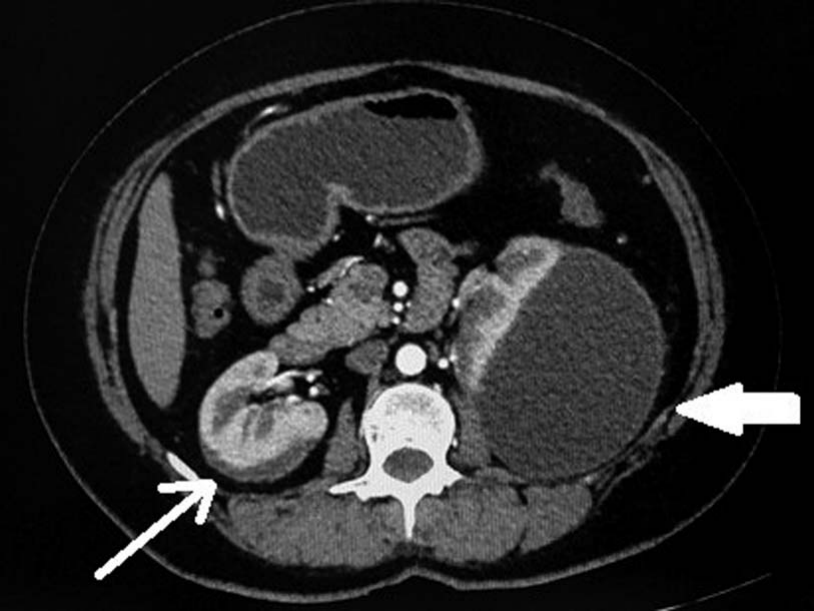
Axial contrast computed tomography shows a well-defined subcapsular collection with thin peripheral enhancement (shown by white arrow). The minimal subcapsular collection is also seen on the right kidney (shown by a thin white arrow).
